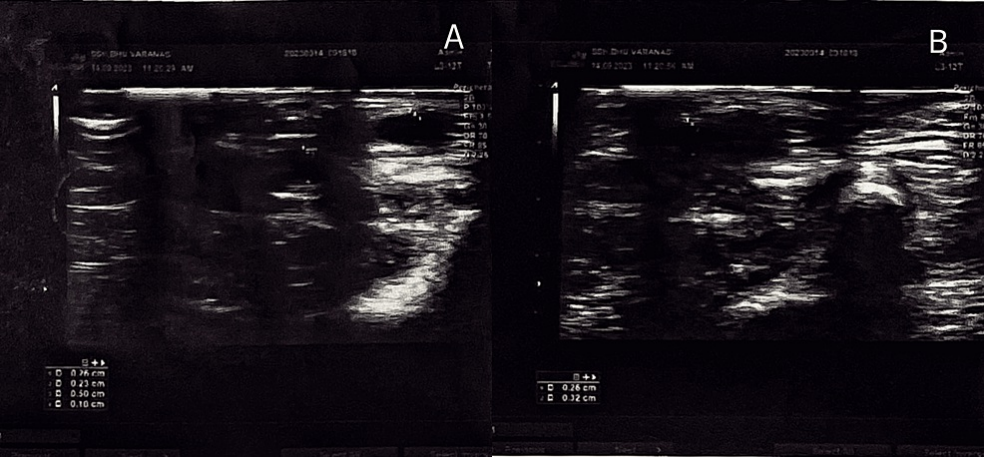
A bilateral upper limb color Doppler film showing blood flow in the (A) right upper limb and (B) left upper limb
